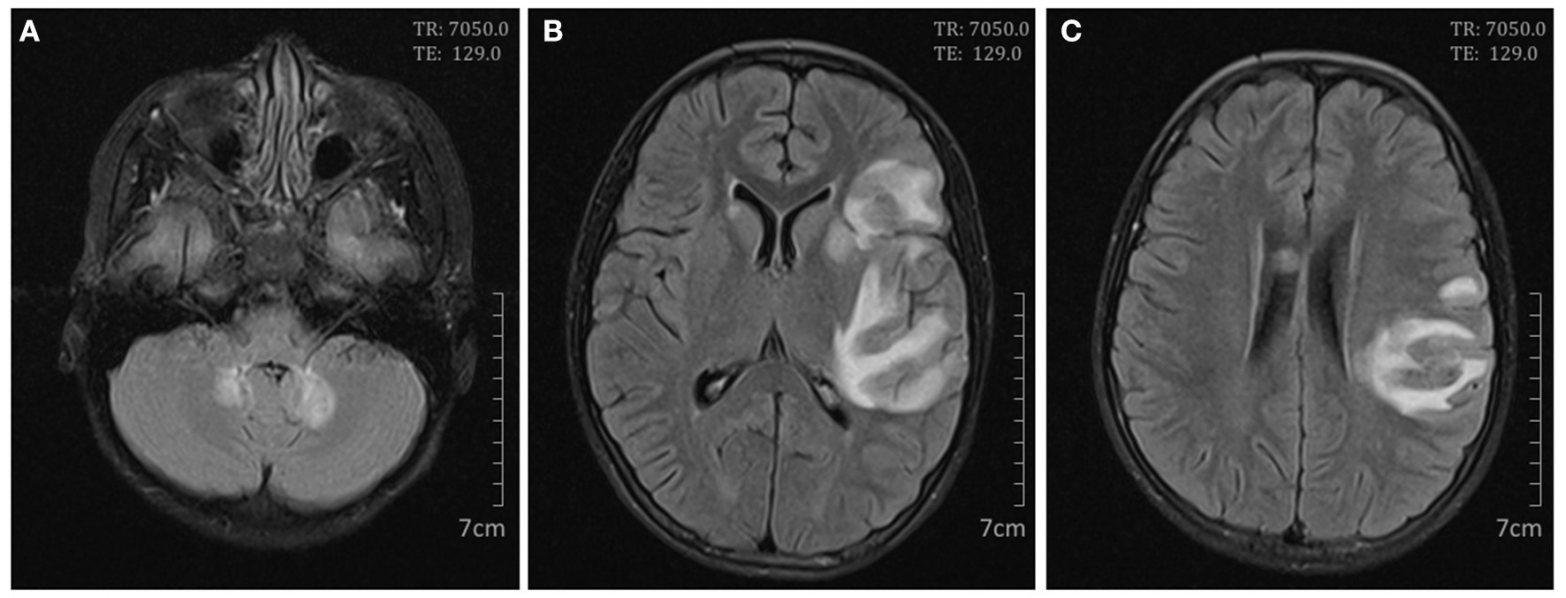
Cerebral magnetic resonance imaging on day 9. Axial FLAIR scan showed high signals in the bilateral cerebellum (A); the bilateral basal ganglias and left frontal, temporal, insular, and parietal lobes (B); and the right corpus callosum and left parietal lobe (C).
